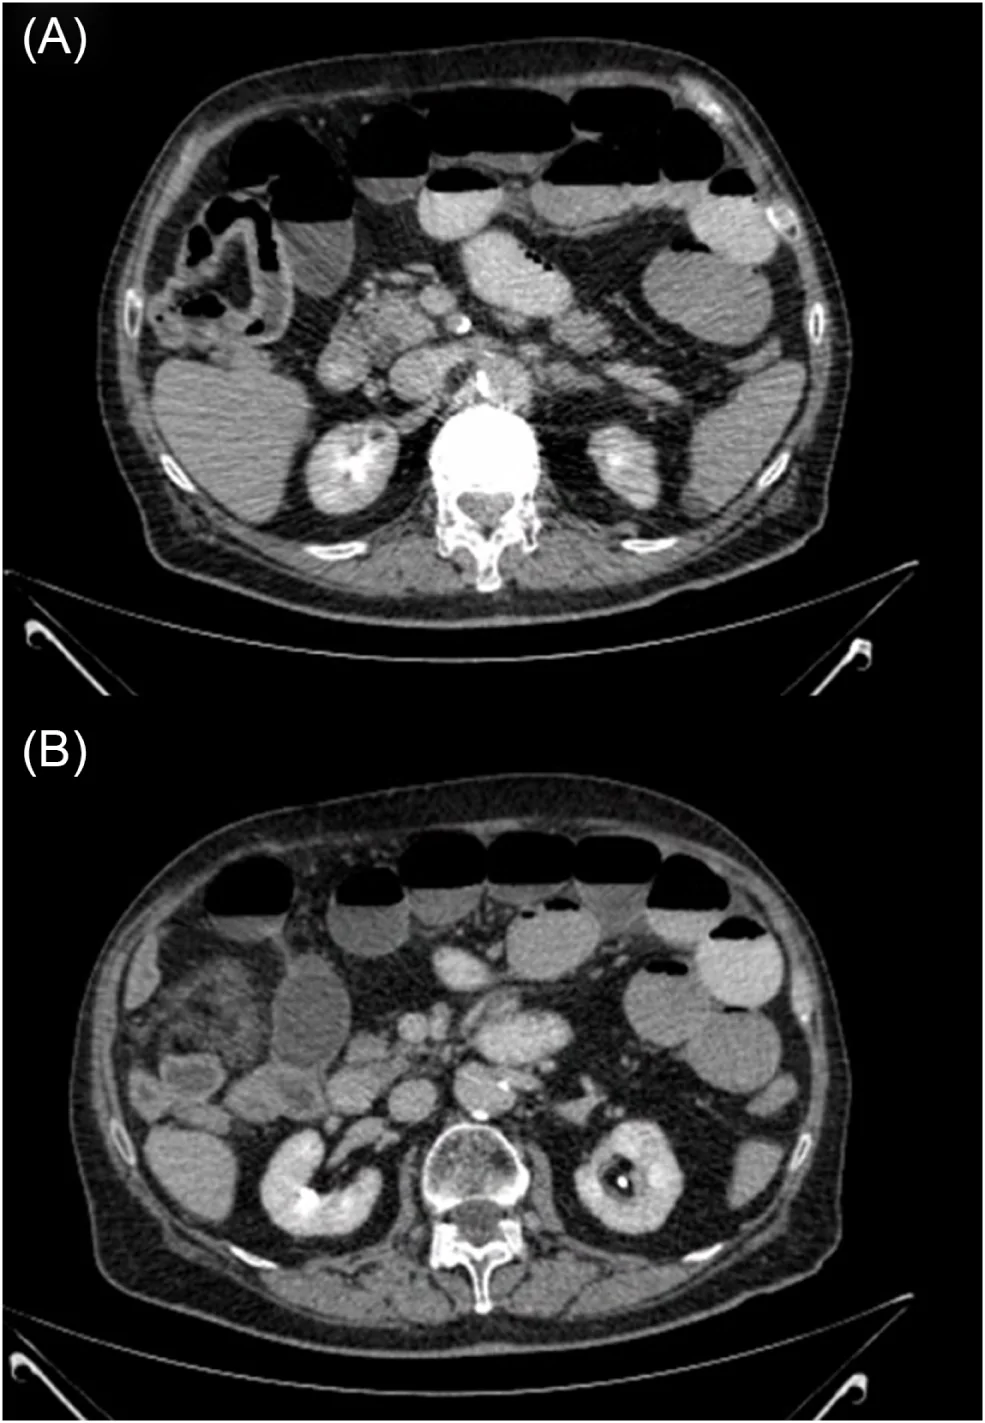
(A and B) Abdominopelvic computed tomography with oral and intravenous contrast showing small bowel distension associated with fat stranding and caliber change at the level of the right hypochondrium with proximal distension and diffuse parietal thickening.
